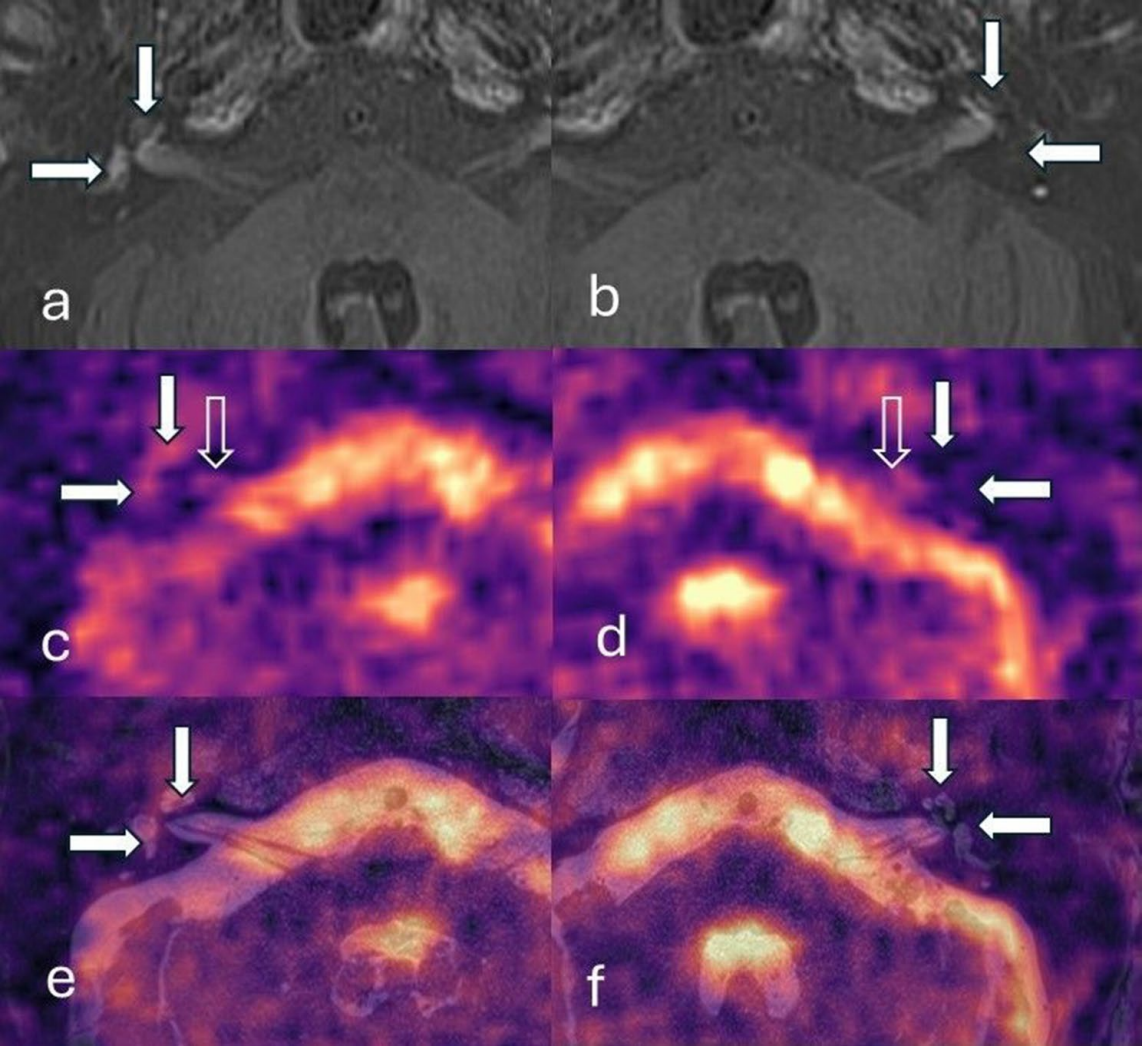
Delayed post-gadolinium 3D inversion-recovery (a,b), unfused ^23^Na MRI images (c,d) and ^23^Na MRI images registered/fused to ^23^Na MRI- T2-SPACE images (e,f). Participant a with left sided severe vestibulo-cochlear endolymphatic hydrops and suboptimal quality ^23^Na MRI images. The endolymphatic hydrops was only correctly lateralised by observer 1 on ^23^Na MRI images after it was registered and fused to structural imaging. The cochlea (vertical filled arrow) and vestibule (horizontal filled arrow) are indicated. Delayed post gadolinium 3D inversion-recovery sequence demonstrates the enhancing perilymphatic space with small non-enhancing endolymphatic structures in the normal right ear (a) whilst there are dilated endolymphatic structures in the pathological left ear with endolymphatic hydrops (b). Axial ^23^Na MRI images and registered/fused ^23^Na MRI- T2-SPACE images through the right ear (c and e) and left ear (d and f). The cochlea (vertical filled arrow) and vestibule (horizontal filled arrow) are indicated on the registered/fused ^23^Na MRI- T2-SPACE images (e, f) and their expected locations are indicated on ^23^Na MRI images (c, d). The normal right ear (c) demonstrated ^23^Na MRI grade 3 signal lateral to the internal auditory meatus (vertical open arrow) but the anatomical compatibility of this signal was uncertain and scored as grade 1 (minor overlap). However, following registration and fusion (c) there was noted to be major overlap with the inner ear structures. The pathological EH left ear (b and d) did not demonstrate any visible ^23^Na MRI inner ear signal (grade 0) lateral to the internal auditory meatus (vertical open arrow). Note the ^23^Na MRI signal from the inferior temporal lobes which lies anterior to the cochlea on each side. The ^23^Na MRI images grey scale imaging may be accessed in the supplementary data
